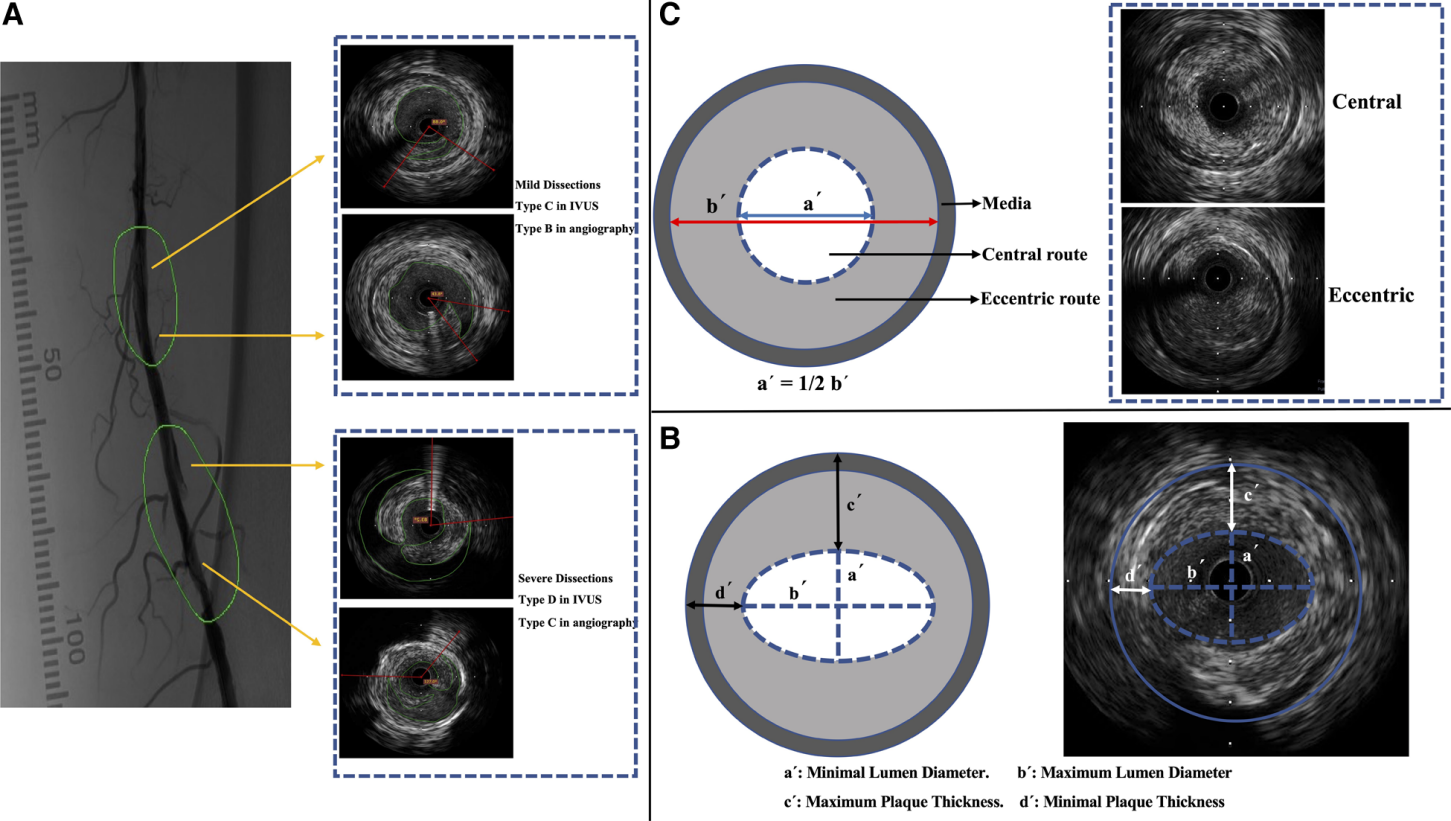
IVUS imaging and characterization. (A) Classification of dissections in IVUS images (according to the classification by Honye et al.) (14) and in angiography (according to the National Heart, Lung, and Blood Institution classification) (15). (B) Measurement of lumen diameter and plaque thickness. (C) Definition of guidewire route. IVUS, intravascular ultrasound.
